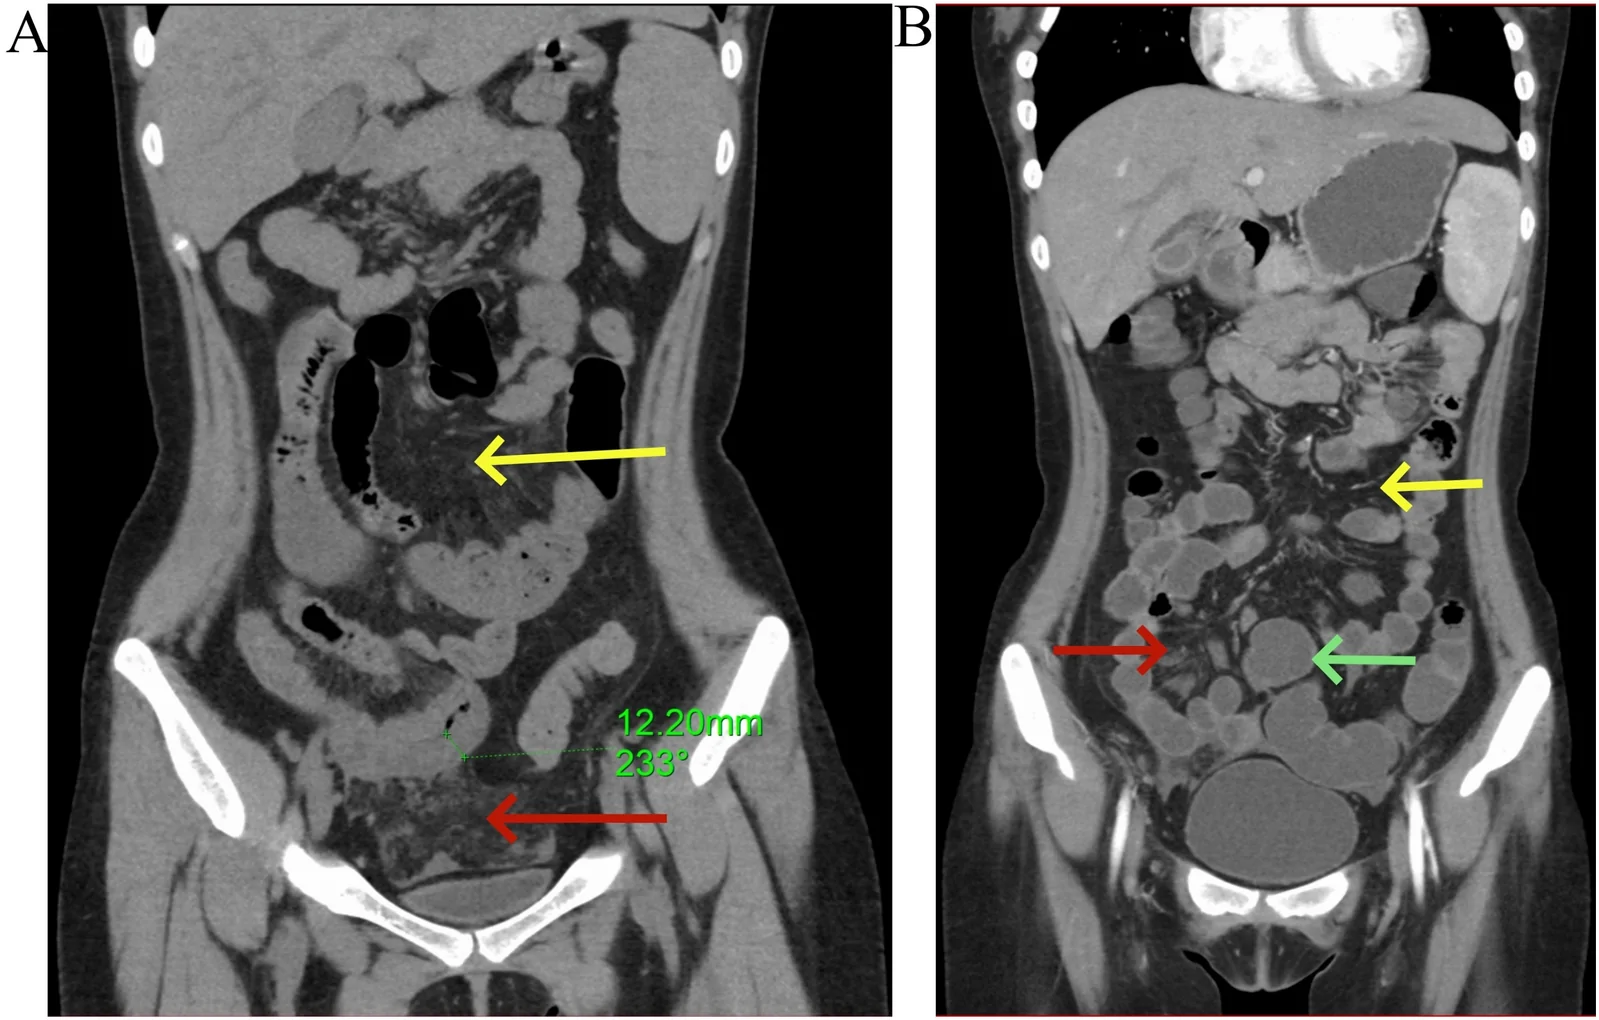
Serial abdominal computed tomography (CT) images. (A) Non-contrast CT of the lower abdomen and pelvis obtained on December 30, 2025, at initial presentation, showing engorged mesenteric vessels (yellow arrows), segmental small-bowel wall thickening measuring up to 12.20 mm (green caliper line), and surrounding fat stranding (yellow arrows). (B) Contrast-enhanced CT enterography performed on January 10, 2026, after pulse methylprednisolone and intravenous immunoglobulin (IVIG) therapy, demonstrating significant improvement in mesenteric vascular engorgement (yellow arrows), small-bowel wall thickening (green arrows), and perienteric fat stranding (yellow arrows) compared with the baseline scan. The overall findings indicate a favorable radiological response to induction therapy.
